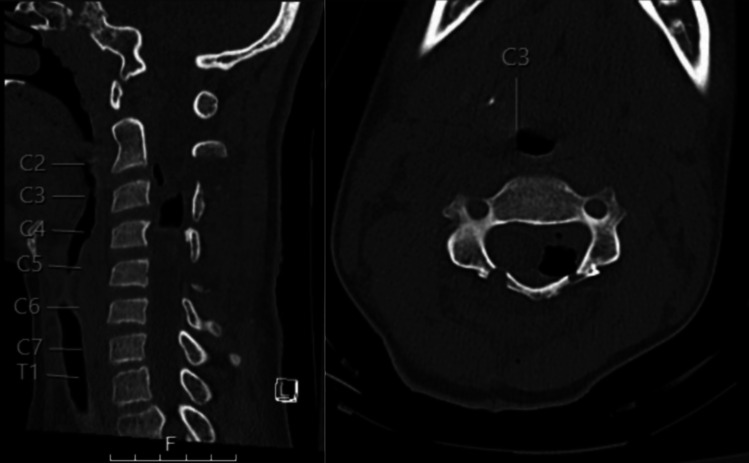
Postoperative non-contrast CT of the cervical spine (sagittal and axial views) displaying gross total resection with expected pneumocephalus and re-expansion of spinal cord within the canal
